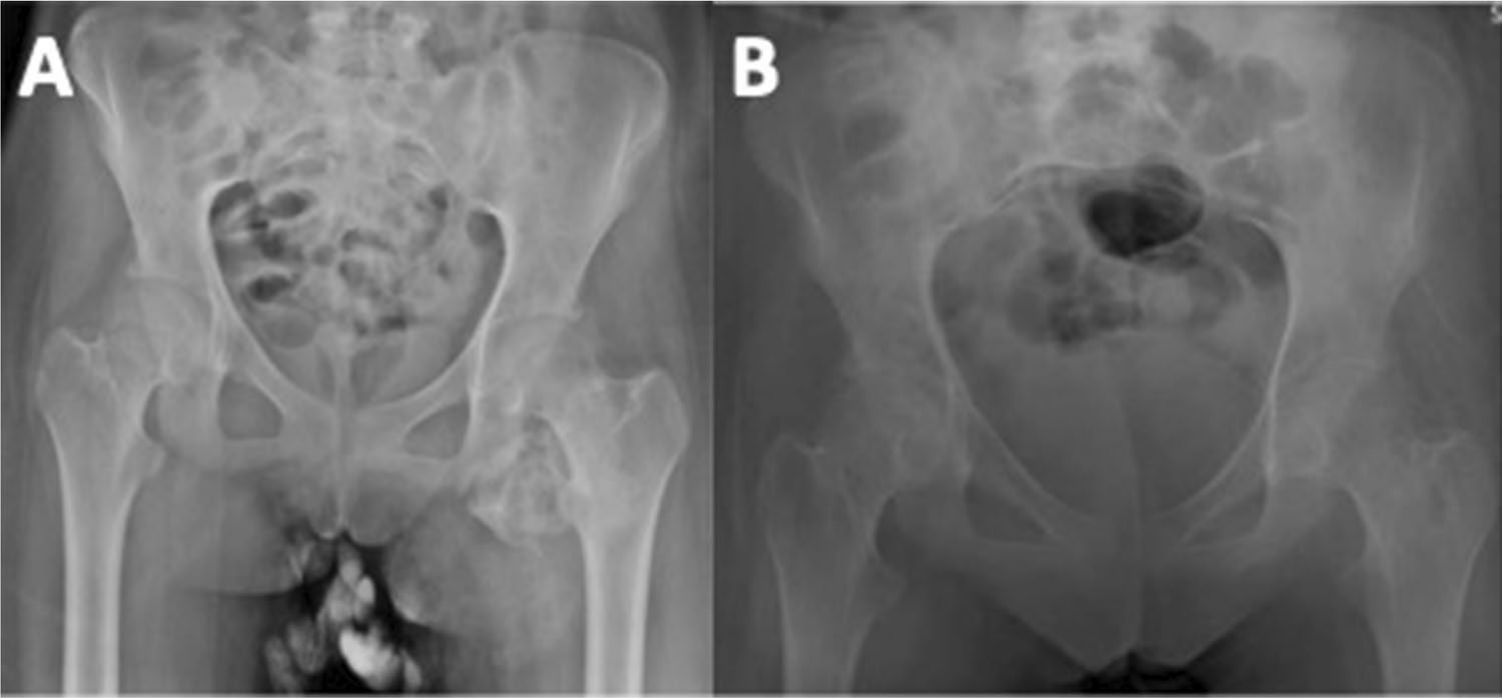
A AP pelvis radiographs demonstrating left hip heterotopic ossification in a 17-year-old female who 4 months prior had been involved in a motor vehicle accident suffering severe traumatic brain injury and B AP pelvis radiographs taken of the same patient in Fig. 2a after operative heterotopic ossification wide resection
